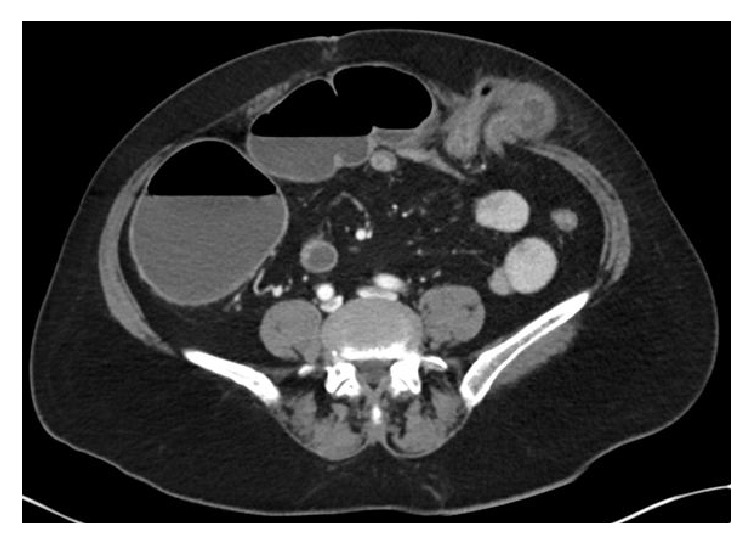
Axial CT showing incisional hernia in the left lower quadrant containing transverse colon causing large bowel obstruction.
